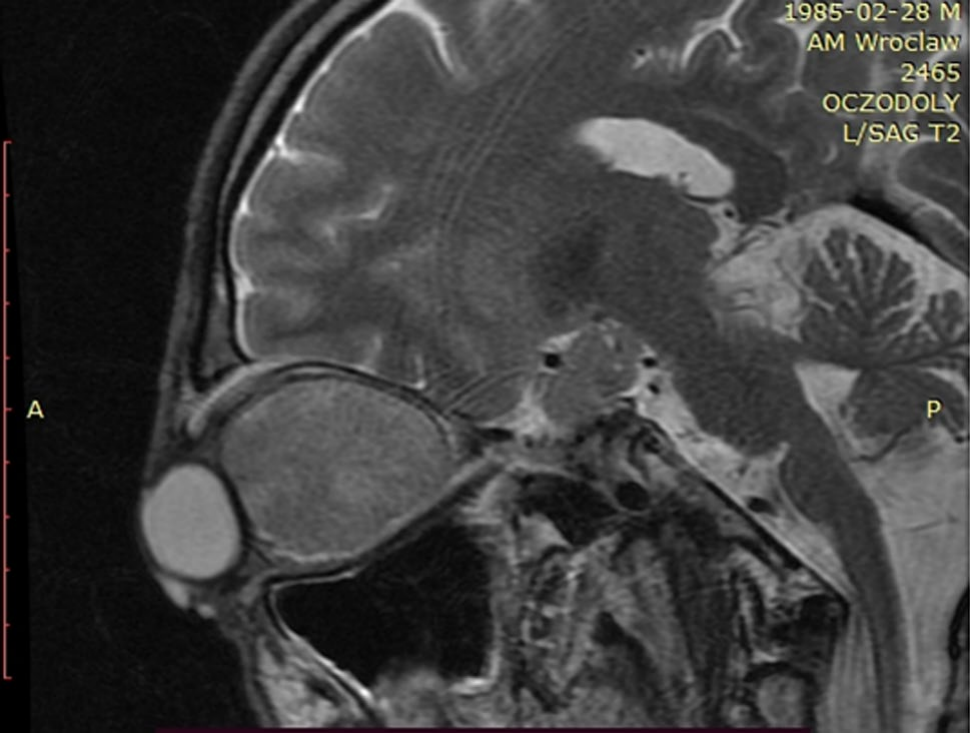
MRI in 2021r, dimensions 43 × 35 × 34 mm
